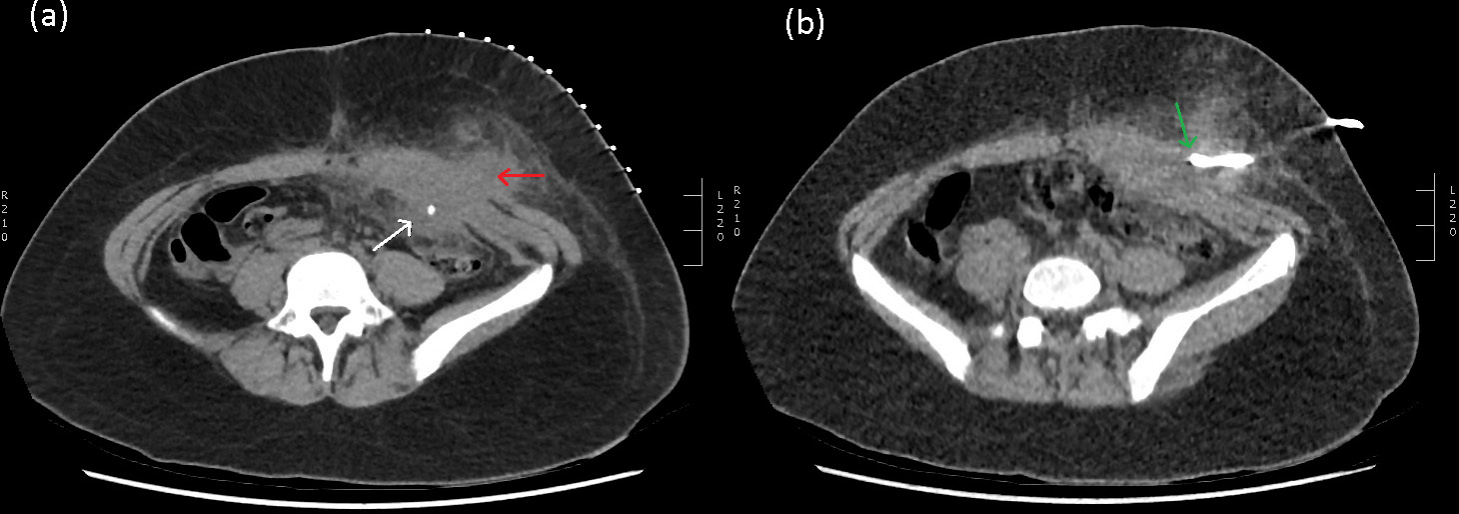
(a) Axial CT slice 3-month post op demonstrating an enlarged
